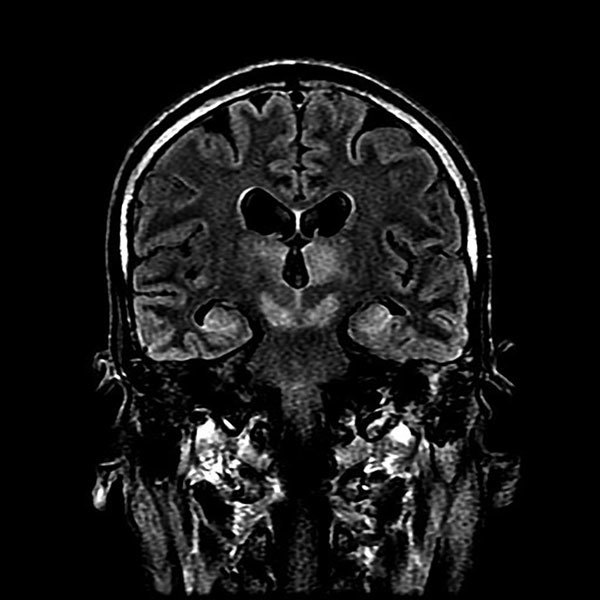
Magnetic resonance imaging of brain of index patient 66 days after double lung transplantation, Hong Kong, China. Coronal FLAIR (FLuid Attenuation Inversion Recovery sequence) image of the head at the level of the lateral ventricles, thalamus, and midbrain shows high signal at bilateral thalamus, midbrain, and medial temporal lobes.
